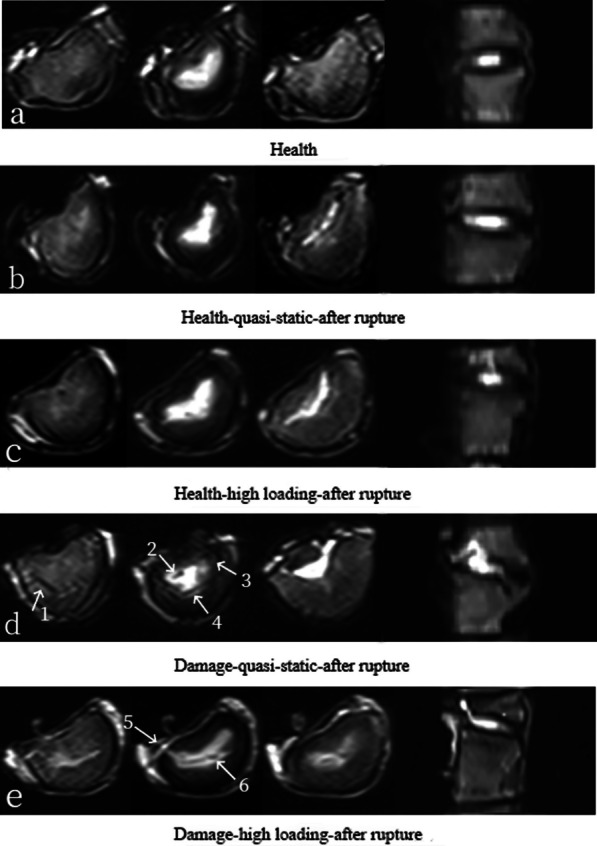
MRI pictures of different moving segments
